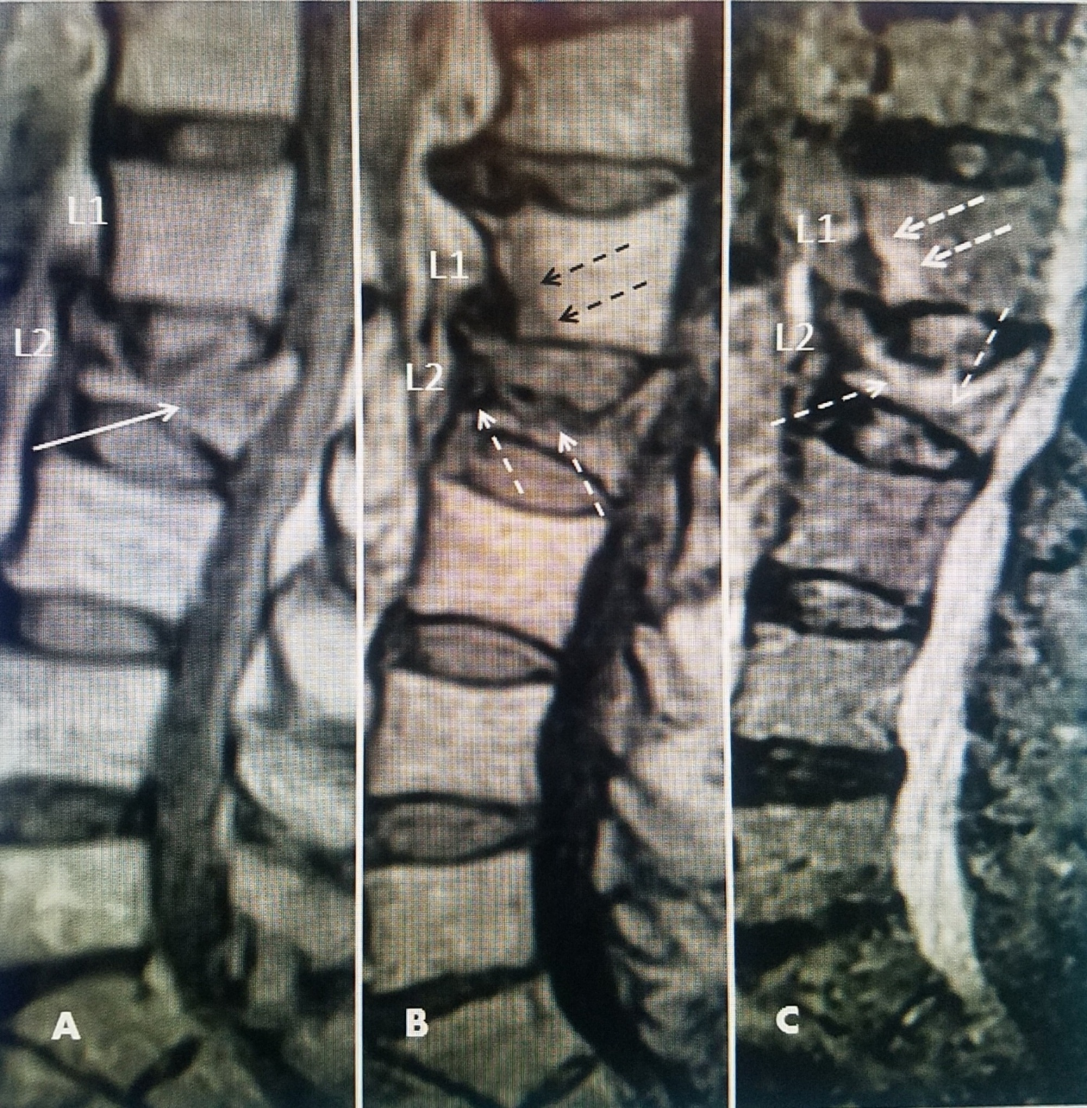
Magnetic resonance imaging (MRI) showing the development of adjacent fractures over seven months A 73-year-old female with a history of multiple falls. She complained of chronic lumbar pain and the original MRI showed an L2 fracture but then developed an L1 adjacent-level fracture over six months despite the use of a brace and anti-inflammatory medication. A: Six weeks after the latest fall, sagittal T1 MRI showing a non-edematous superior endplate fracture at L2 (solid white arrow) with the posterior displacement of the superior endplate into the spinal canal. B; Sagittal T1 MRI taken five months later shows progressive collapse at L2 with slight edema under the superior endplate (dashed white arrows) and new edema in the anterior inferior edge of L1 (dashed black arrows). C: MRI with short TI inversion recovery (STIR) images five months after initial MRI now showing extensive edema involving almost the entire body of L2 (thin dashed white arrow) and a clear image of new edema in the anterior inferior part of L1 (thicker dashed white arrows).
